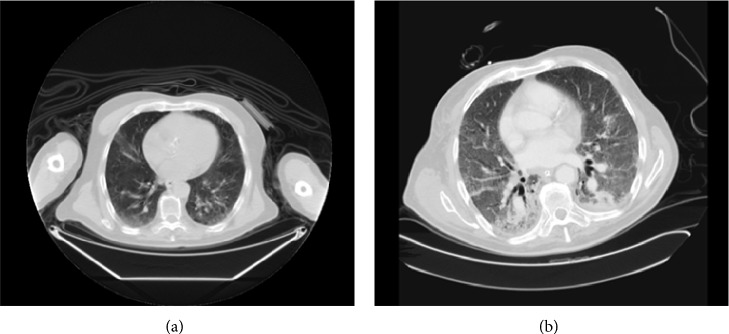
(a) Chest CT scan of patient 2, three months prior to development of respiratory symptoms. (b) Chest CT scan of patient 2, at the beginning of respiratory symptoms: diffuse interstitial infiltrates shown in both lungs.
